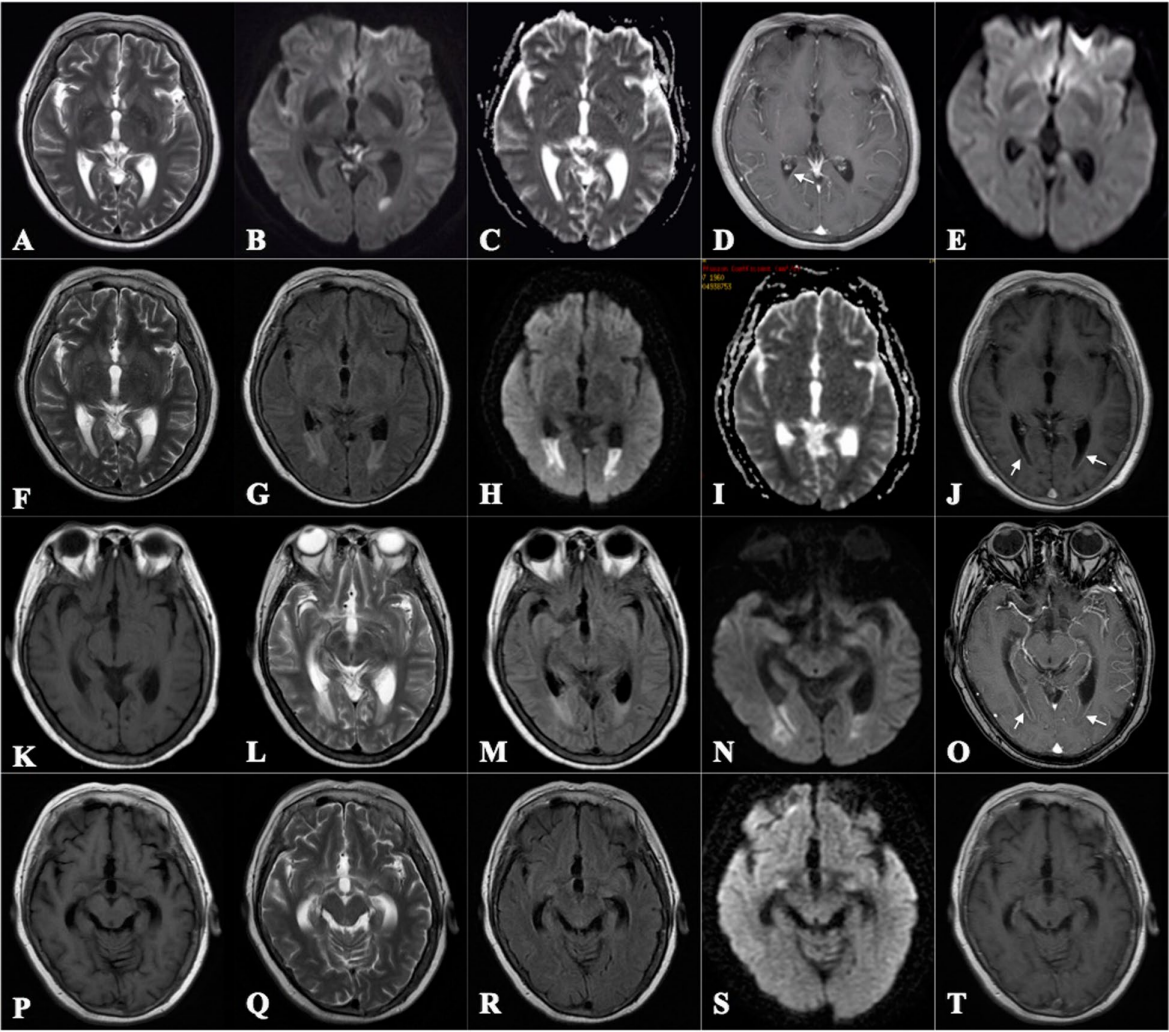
Brain magnetic resonance imaging performed at the previous hospital and our hospital. For the previous hospital, images at the time of admission (A–D) and 49 days after admission (E) are shown. For our hospital, images at the time of admission (F–J) and 25 (K–O), and 42 (P–T) days after admission are shown. Ventricular debris appeared as hypointense areas in the cerebrospinal fluid (CSF) on T2-weighted (A) and apparent diffusion coefficient (ADC) (C) images and as hyperintense areas in the CSF on the corresponding diffusion-weighted image (DWI) of the left occipital horn (B). Slight ependymal enhancement was found in the right occipital horn on the enhanced T1-weighted image (D). The hyperintensity in the left occipital horn was no longer apparent on DWI (E). T2-weighted (F) and ADC (I) images showed decreased intensity in the CSF in the occipital horn, whereas the corresponding fluid attenuation recovery (G) and DWI (H) images showed increased intensity with an enhanced ventricular line (arrow, J). The abnormal intensity in the bilateral occipital horns was reduced (K–N) but the ependymal enhancement was still obvious (arrow, O). The abnormal intensity had disappeared (P–S) and no enhancement was observed (T)
